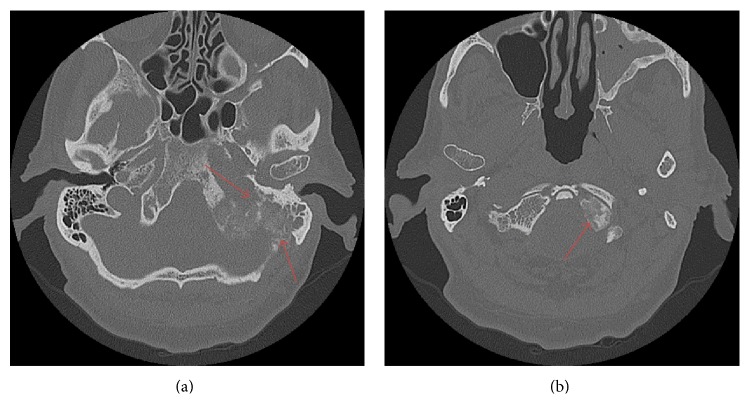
CT scan of the head without contrast, axial images, 8/11/2014, showing a destructive lesion centered within the left occipital bone with extension into the inferior mastoid temporal bone (a) and occipital condyle (b).
